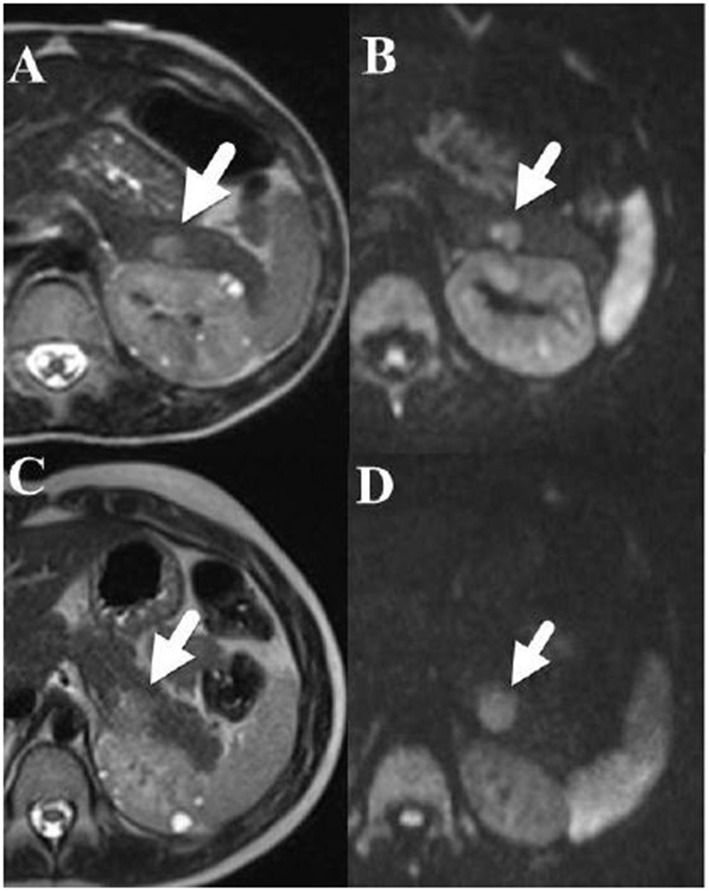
(A) [Upper left] Axial T2-weighted fast spin echo and (B) [Upper right] diffusion weighted MRI images performed in a 6-year-old boy show a 1.2 × 0.8 cm hyperintense mass (arrow) in the tail of the pancreas that restricts diffusion. Note that small cysts are also present within the left kidney. (C) [Lower left] an Axial T2-weighted fast spin echo and (D) [Lower right] diffusion weighted MRI images performed 3 years later show that the pancreatic tail mass (arrow) has grown slowly and now measures 1.4 × 1.5 cm.
